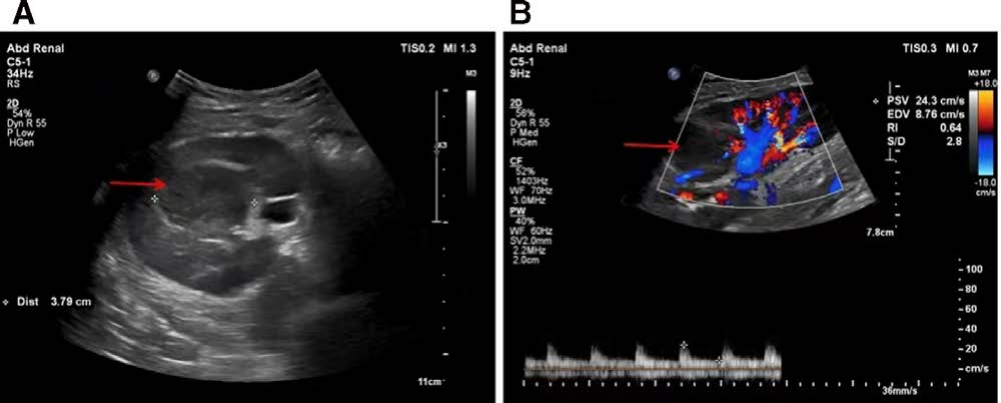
(A–B) Color Doppler ultrasound depicted a hypoechoic lesion of 4.3 × 3.8cm with no blood flow signals inside in the renal graft (arrow).
